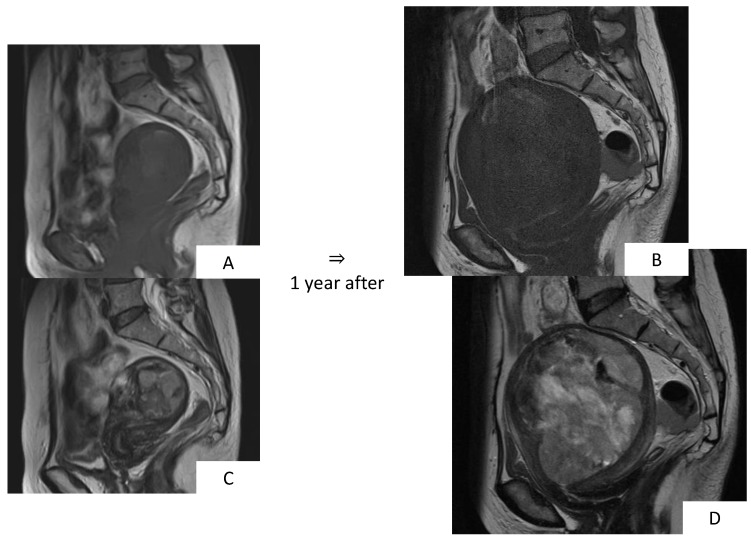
Magnetic resonance images of leiomyosarcoma. (A,B): T1-weighted sagittal sections, (C,D): T2-weighted sagittal sections. In the myometrium, in T2-weighted images, overall, there is high signal intensity with ill-defined borders; in T1-weighted images, there is mass accompanied by internal hemorrhage. Follow-up observation was selected for this case, with the diagnosis of uterine sarcoma 1 year thereafter.
